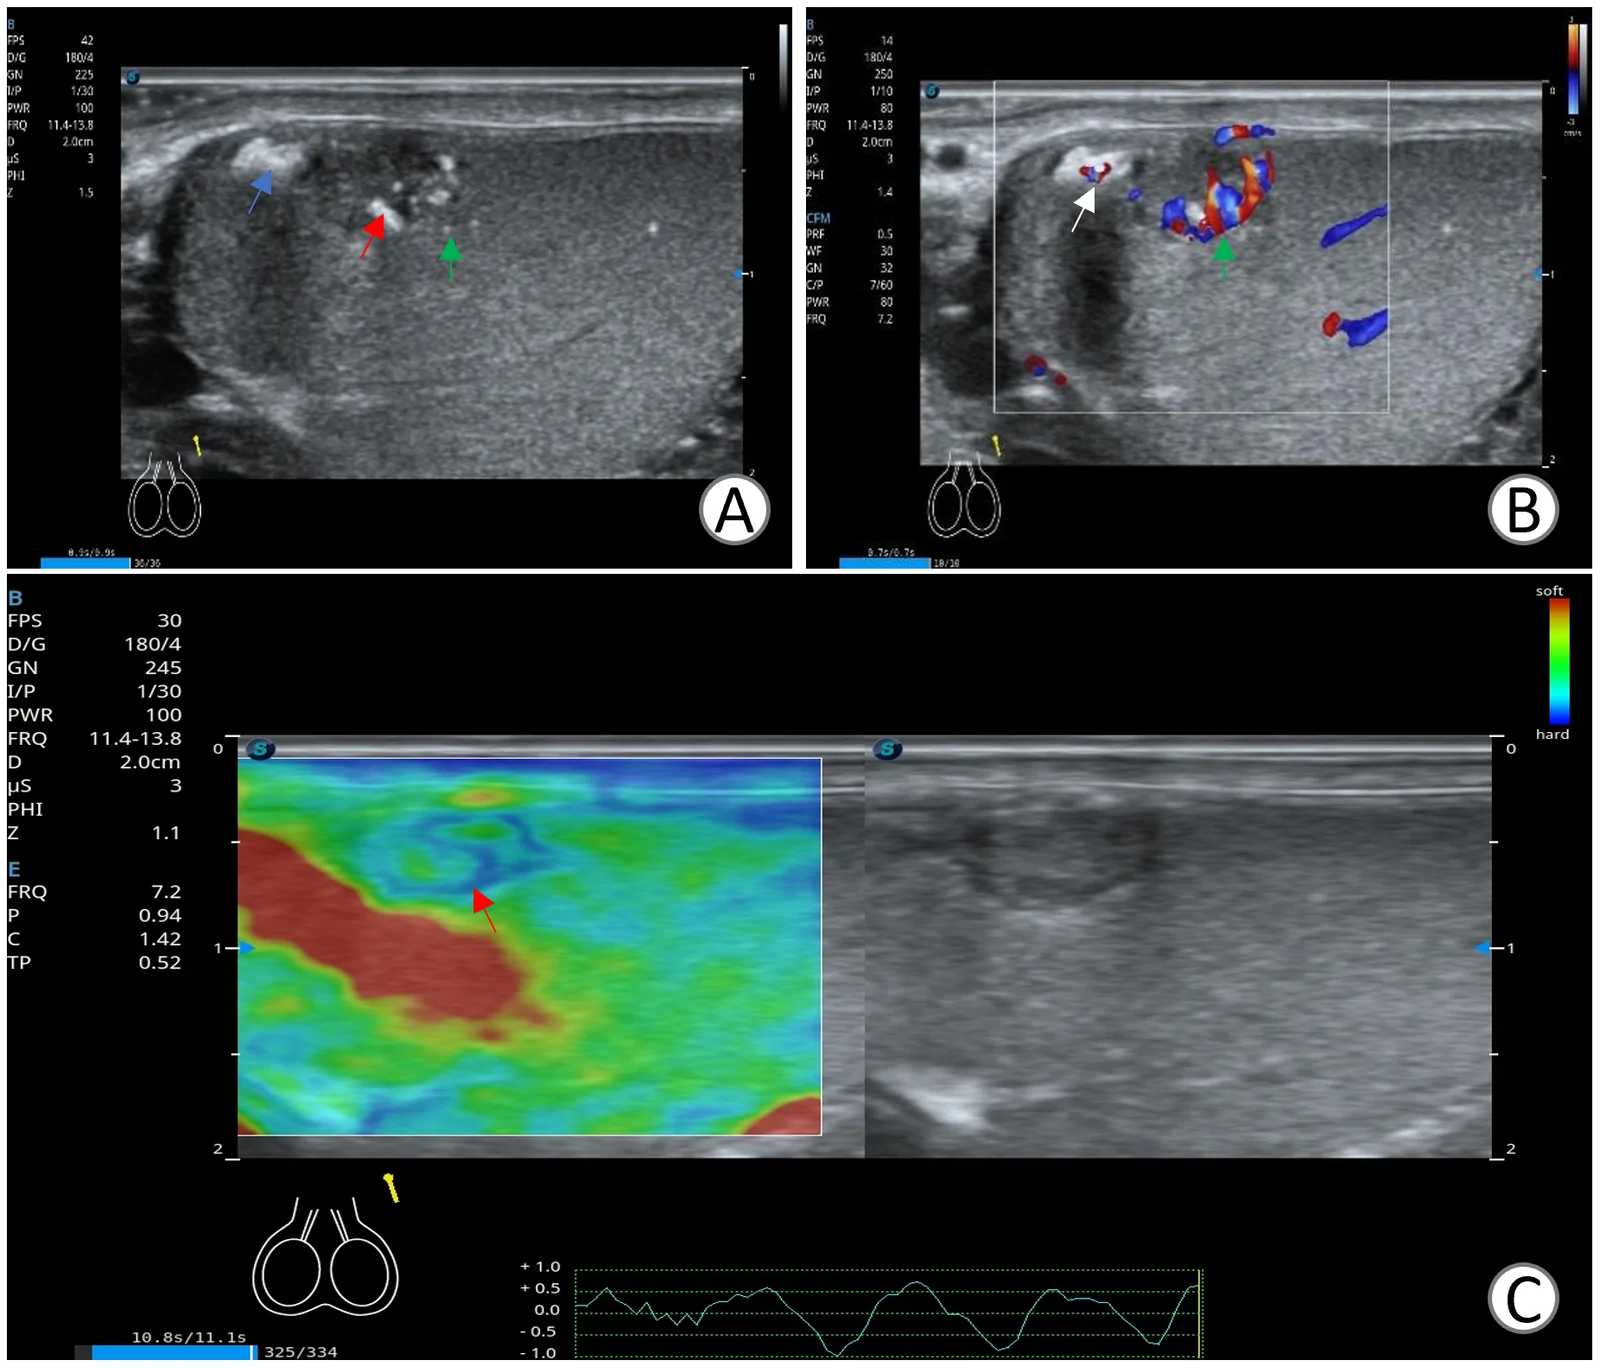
Ultrasonographic features of primary testicular intestinal-type adenocarcinoma. (A) Grayscale ultrasound shows an irregular, poorly marginated hypoechoic nodule in the upper-to-middle portion of the left testis. Peripheral echogenic foci, including punctate (green arrow), linear (red arrow), and clustered (blue arrow) types, are visible at the lesion margin, with posterior acoustic shadowing. (B) Color Doppler flow imaging demonstrates twinkle artifact posterior to the echogenic foci (white arrow), as well as abundant peripheral vascularity, with vessels extending into the lesion and forming a compact “ball-like” vascular configuration (green arrow). (C) Strain elastography shows increased stiffness at the lesion periphery.
